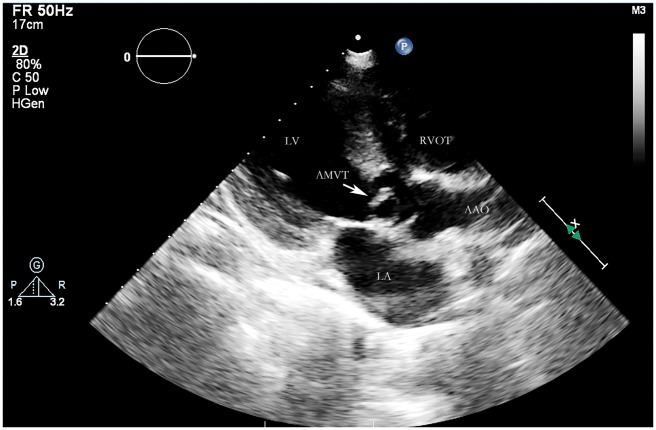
The parasternal long-axis view showed a fixed accessory mitral valve tissue attached to the anterior leaflet, which caused left ventricular outflow tract obstruction.
